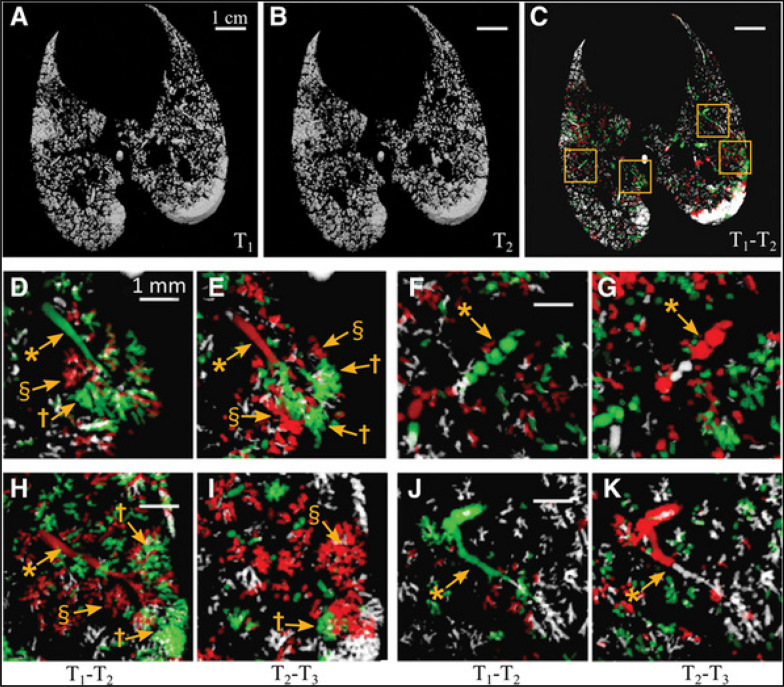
In vivo recruitment/derecruitment of airspaces characterized by phase-contrast CT. A–E Alveolar recruitment/derecruitment (R/D) occurs alternately in neighboring lung units over short time scales ~ 1 min, in injured lung at positive end-expiratory pressure (PEEP) 6 cm H2O. A–C 3D renderings of aerated lung regions obtained by segmentation of synchrotron phase-contrast CT images at 0 (A) and 84 s (B) in a 2.5 mm thick slice, in injured lung at PEEP 6 cm H_2_O; C, R/D map is quantified using image registration between T1 (A) and T2 (B). White: aerated, no change; black: nonaerated, no change; green: opening; and red: closing. Yellow squares delineate regions of interest magnified in D–E with D, F, H, and J computed from two successive images acquired at 0 and 84 s (T1–T2) and E, G, I, and K from the subsequent time interval between 84 and 159 s (T2–T3). *Bronchioles, †recruiting airspaces, and §derecruiting airspaces. Reproduced with permission from [41]
